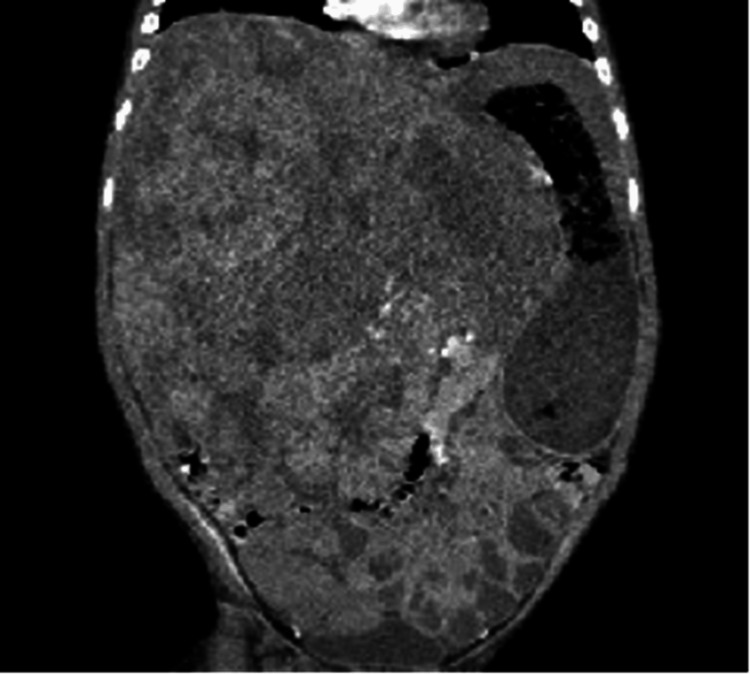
Original non-contrast CT scan demonstrating mass effect of the patient’s hepatoblastoma. CT: computed tomography
